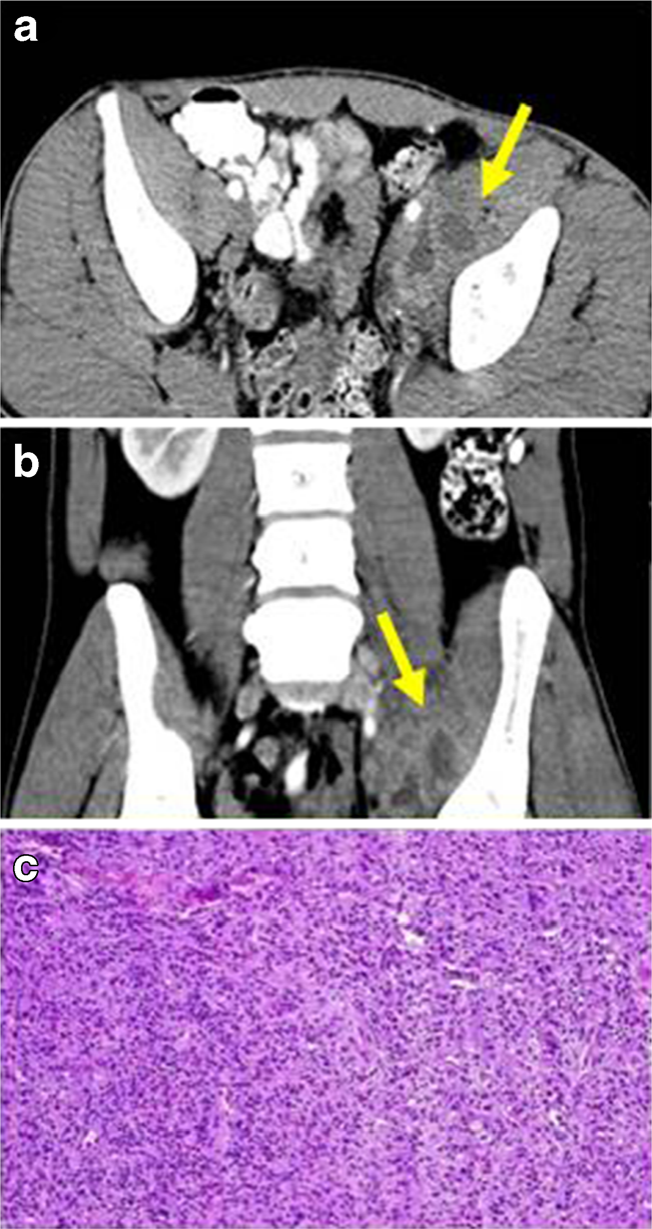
PATHOLOGY: IMT of the iliopsoas muscle. a Axial and b coronal reformations of contrast-enhanced CT where an ill-defined and heterogeneous mass encompassing iliopsoas muscle and external iliac vessels, mimicking malignant sarcomatous neoplasm was observed. c Sample of the lesion obtained after surgical excision of the mass. Proliferation of fibroblast with a diffuse infiltration of lymphocytes, eosinophils and macrophages were found. No mitotic figures or cellular atypia were present. The mass was well-defined and no signs of invasiveness were found
